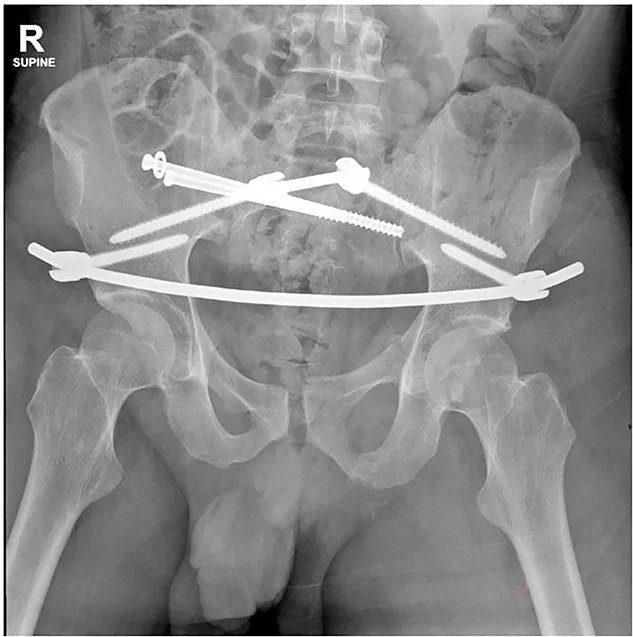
Post-surgical pelvic radiograph demonstrating INFIX technique for pelvic fracture, in addition to iliosacral fixation.
